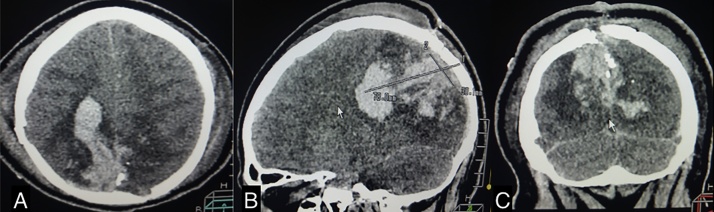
Axial, sagital and coronal non contrasted CT scan that shows intracerebral biparietal posterior haemorrhage.
